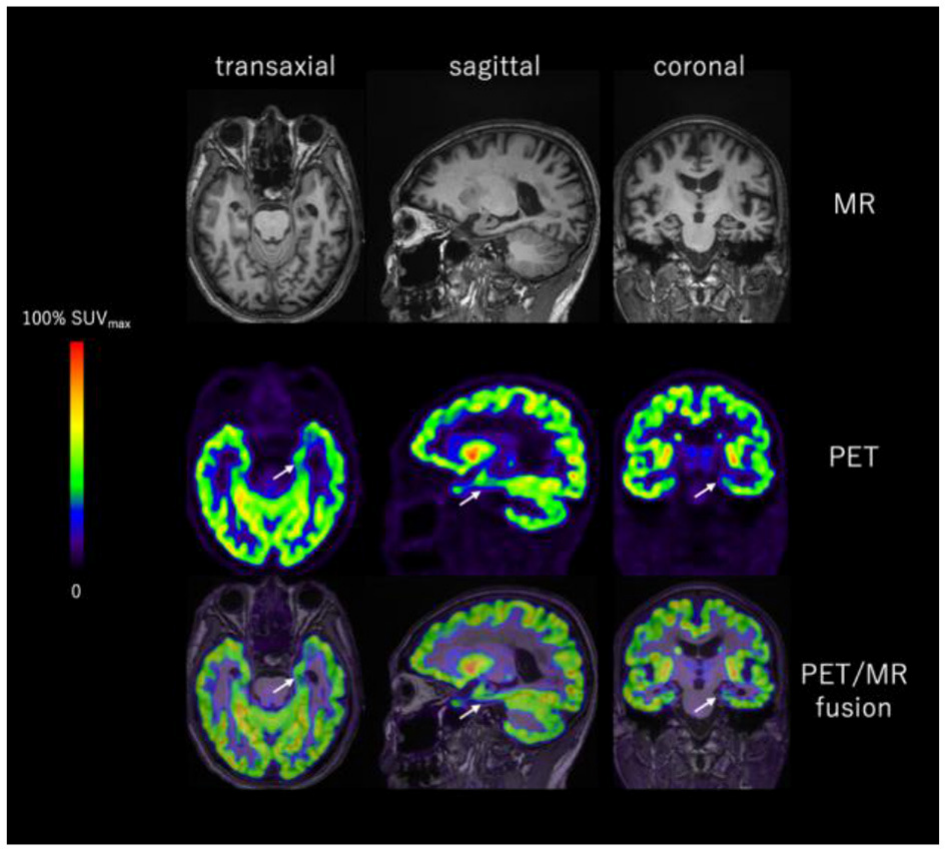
Representative images of T1WI MRI and [^18^F]SynVesT-1 PET of a [^18^F]Florbetapir positive AD patient. SV2A binding is significantly reduced in the left hippocampus (white arrow). Images provided by PET Center, Huashan Hospital, Fudan University and Department of Nuclear Medicine, East Hospital, Tongji University.
